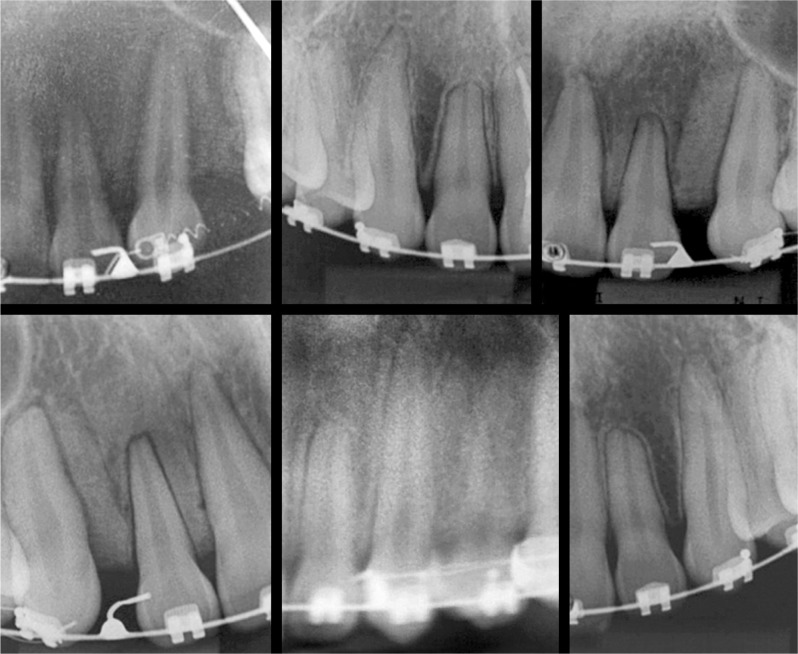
Periapical radiograph of patients comprising the mild to moderate IERR
